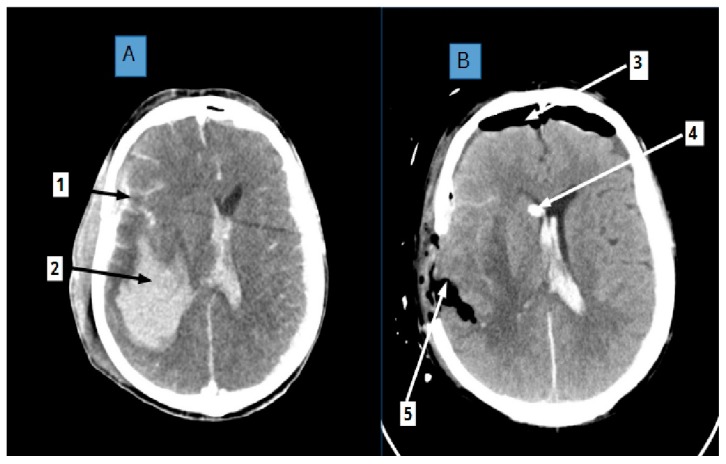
56 years old male, a victim of craniocerebral trauma (CT) due to assault. Assessment upon admission rated him at a 3 GCS (Glasgow Coma Scale) without autonomic respiration. CT A: showing huge intracerebral hematoma in right hemisphere (arrow 2) surrounded with edematous area. Subarachnoid hemorrhage (arrow 1) as well as intraventricular blood clot. Right-sided craniectomy was performed and hematoma evacuated. Intraventricular external drainage was installed. CT B: shows postoperative cavity with air remnants (arrow 5), intraventricular catheter tip in right frontal horn is also seen (arrow 4). Air in subarachnoid space in frontal region is present due to brain volume reduction (arrow 3). Noteworthy is that the shift of medial line structures is less advanced compared with CT A taken before surgery. The patient survived with the GOS (Glasgow Outcome Scale), which might be related to the early application of decompressive techniques e.g., craniectomy and increased CSF outflow causing more adequate perineuronal net geometry. The study was conducted in accordance with the Declaration of Helsinki, and the Bioethical Commission of Pomeranian Medical University in Szczecin, Poland, approved the protocol (permission numbers KB-0012/156/17).
